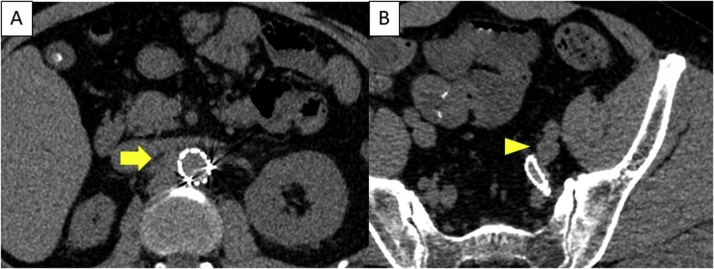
One-year surveillance computed tomography after surgery. (A) No blood leaked from the vessel at the right of the aorta (arrow). (B) The left internal iliac pseudoaneurysm decreased in the size (arrowhead).
